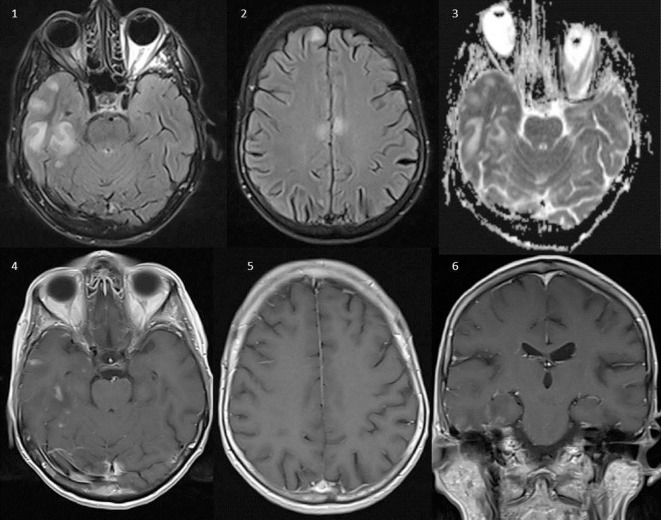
Brain magnetic resonance imaging (Siemens Aera 1.5 T). Axial FLAIR (1, 2), axial ADC-maps (3), axial T1 after intravenous gadolinium (4, 5), and coronal T1 after intravenous gadolinium (6). Several lesions with high T2-signal on FLAIR in the right temporal lobe, bilateral in the frontal lobe, and the callosal body without signs of restricted diffusion on the ADC-maps, compatible with vasogenic edema. Several lesions in the right temporal lobe show patchy contrast enhancement after intravenous administration of gadolinium-based contrast (4–6). None of the lesions were hemorrhagic.
